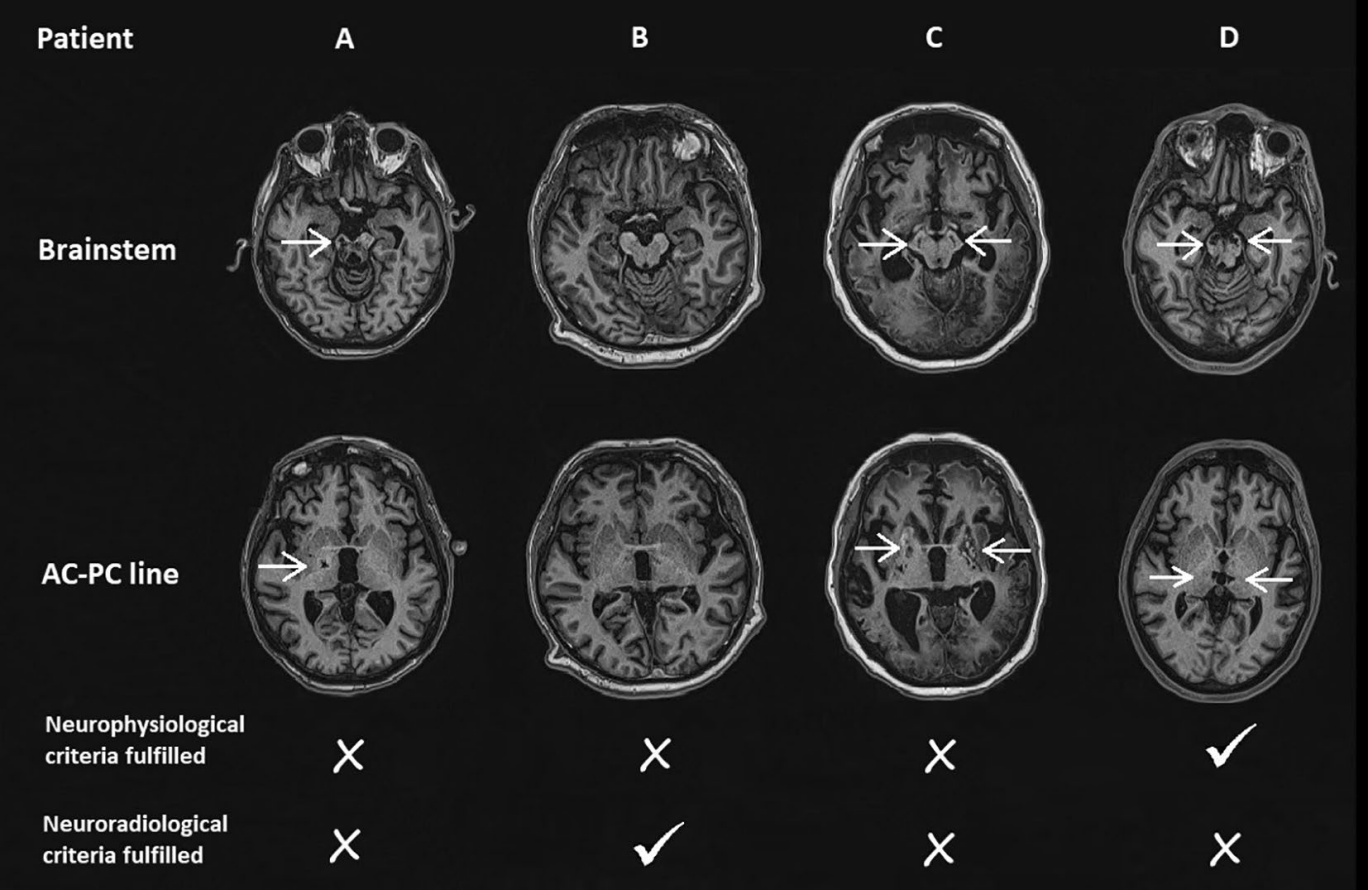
Brain MRIs of patients who weren’t candidates for DBS implantation, T1 MPRAGE, axial plane. (A) Female patient, 51 yrs, DoC due to intracerebral hemorrhage; significant lesion observed at the level of brainstem and thalamus. Patient didn’t fulfil both neurophysiological and neuroradiological criteria. (B) Male patient, 57 yrs, DoC due to cardiac arrest, no significant lesion on MRI. Patient didn’t fulfil neurophysiological criteria. (C) Female patient, 46 yrs, DoC due to cardiac arrest; global ischemic cortical, basal ganglia and brainstem changes. Patient didn’t fulfil both neurophysiological and neuroradiological criteria. (D) Male patient, 36 yrs, DoC due to septic embolization of the brain; significant lesion observed at the level of brainstem and thalamus. Patient fulfil neurophysiological criteria. White arrows represents lesions visible on different levels of brain MRI.
